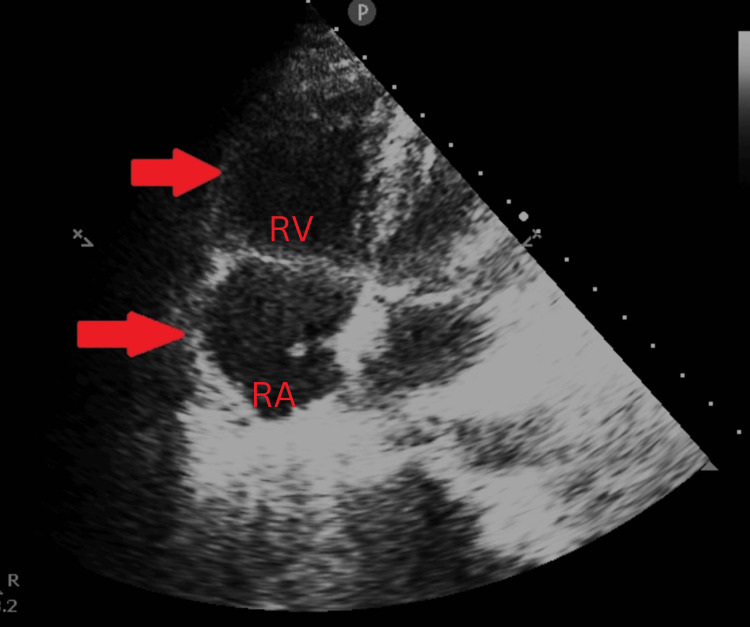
Point of care ultrasound showing the right atrium and right ventricle dilated as shown by red arrows in the apical four-chamber view
